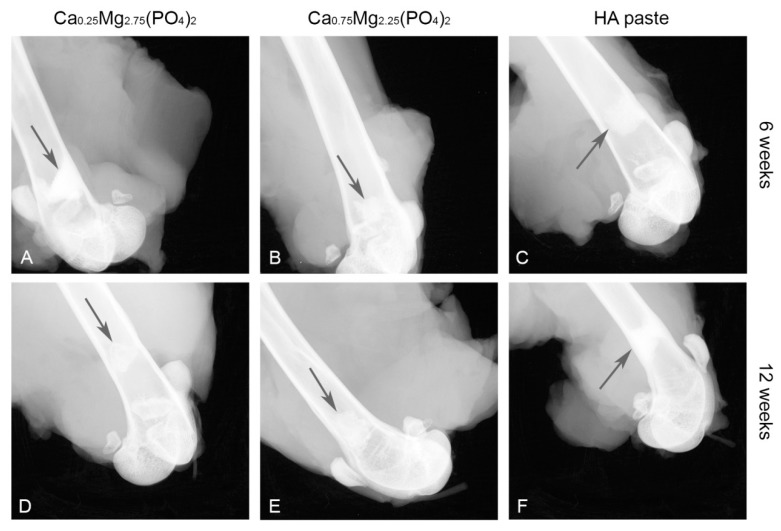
X-ray images of the Ca_0,25_Mg_2,75_(PO_4_)_2_ paste (A,D), the Ca_0,75_Mg_2,25_(PO_4_)_2_ paste (B,E) and HA paste (C,F) in the femoral defect directly after explantation after 6 and 12 weeks. The implants are marked by arrows.
